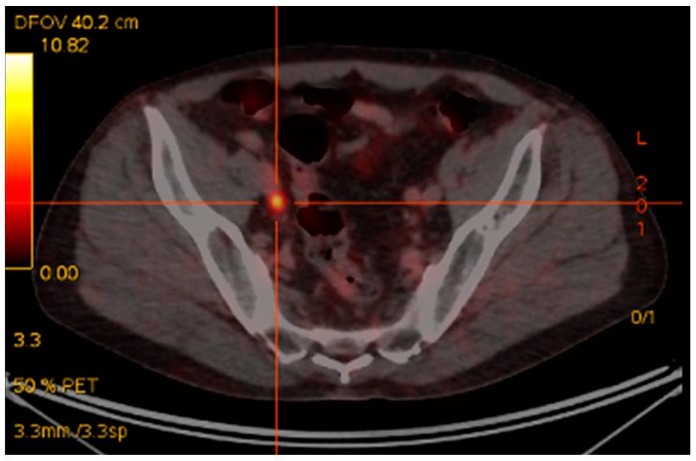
Rising PSA post-prostatectomy. PSA 0.9 ng/mL. CT unremarkable. ^68^Ga-PSMA-avid right pelvic lymph node.
